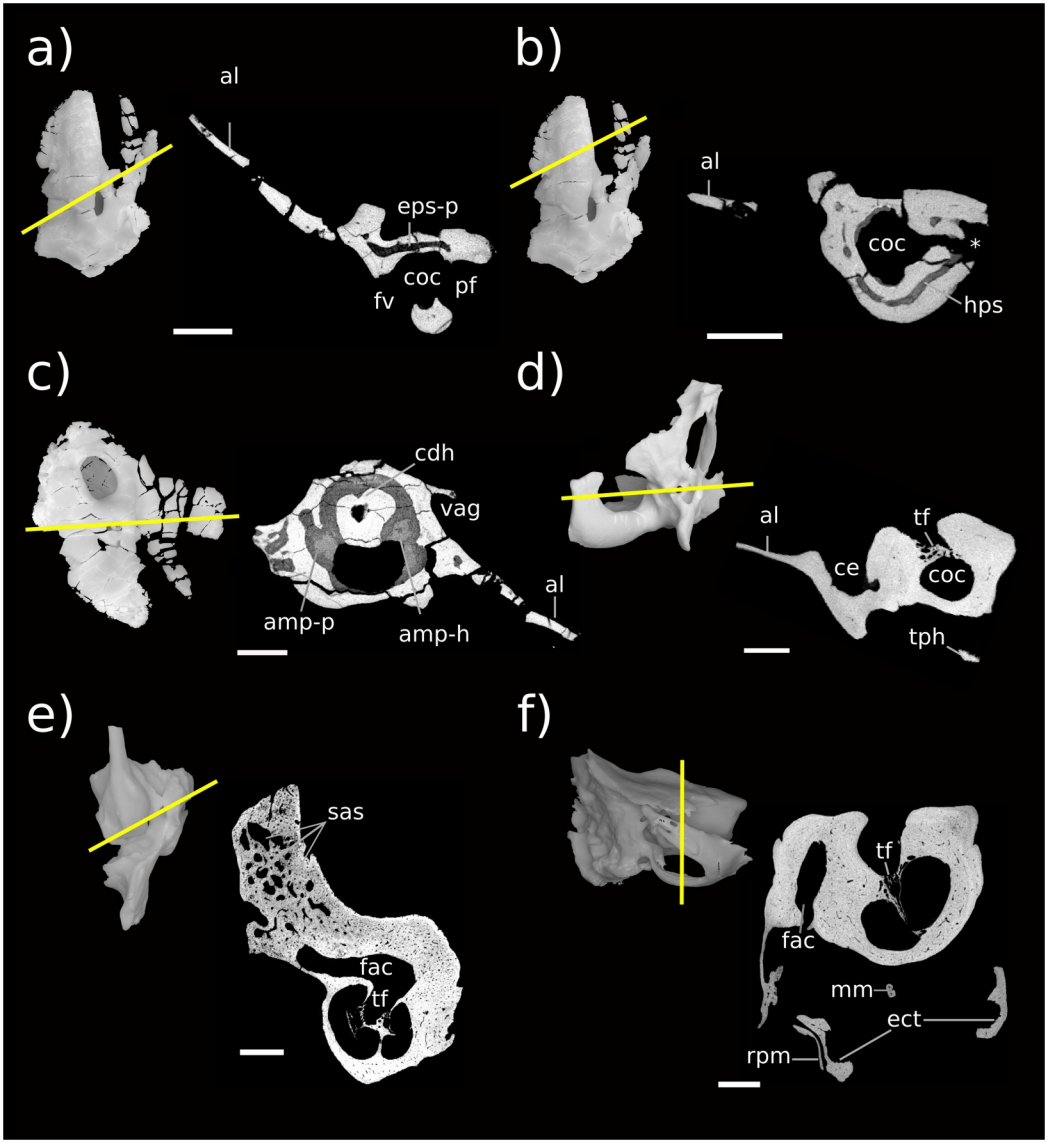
Resliced CT images showing *Priacodon* and several extant mammals. a, b, c images of *Priacodon*; d, coronal section through promontorium of *Ornithorhynchus*; e, coronal section through promontorium of *Didelphis*; f, coronal section through promontorium of *Dasypus*. a, b, are coronal sections through the promontorium of *Priacodon*, a is taken posterior to b to show the posterior epicochlear sinus; b, is taken rostral to the fenestra vestibuli and perilymphatic foramen to show the hypocochlear sinus. c, shows horizontal plane through the horizontal semicircular canal and its centripetal diverticulum. All scale bars are 1 mm; for 2D slices in a, b, d, e, and f lateral is toward the left of the page and dorsal is toward the top of the page; in c lateral is toward the right of the page and posterior is toward the top of the page. Asterisk shows location of damage to anterior wall of perilymphatic foramen in *Priacodon*. Refer to list of abbreviations in text for other abbreviations.
